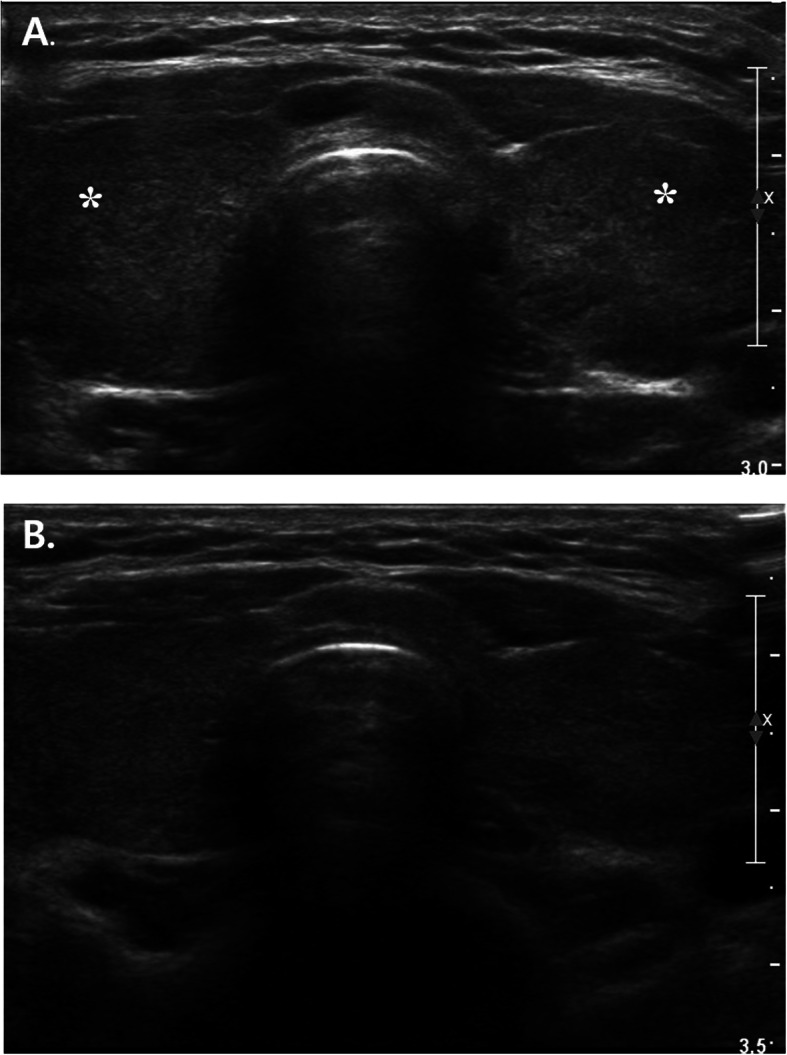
Ultrasonography of thyroid on admission (a) showed more hypoechoic and enlarged thyroid gland (White Asterisk) but 6-month follow-up ultrasonography (b) showed normal sized thyroid gland
